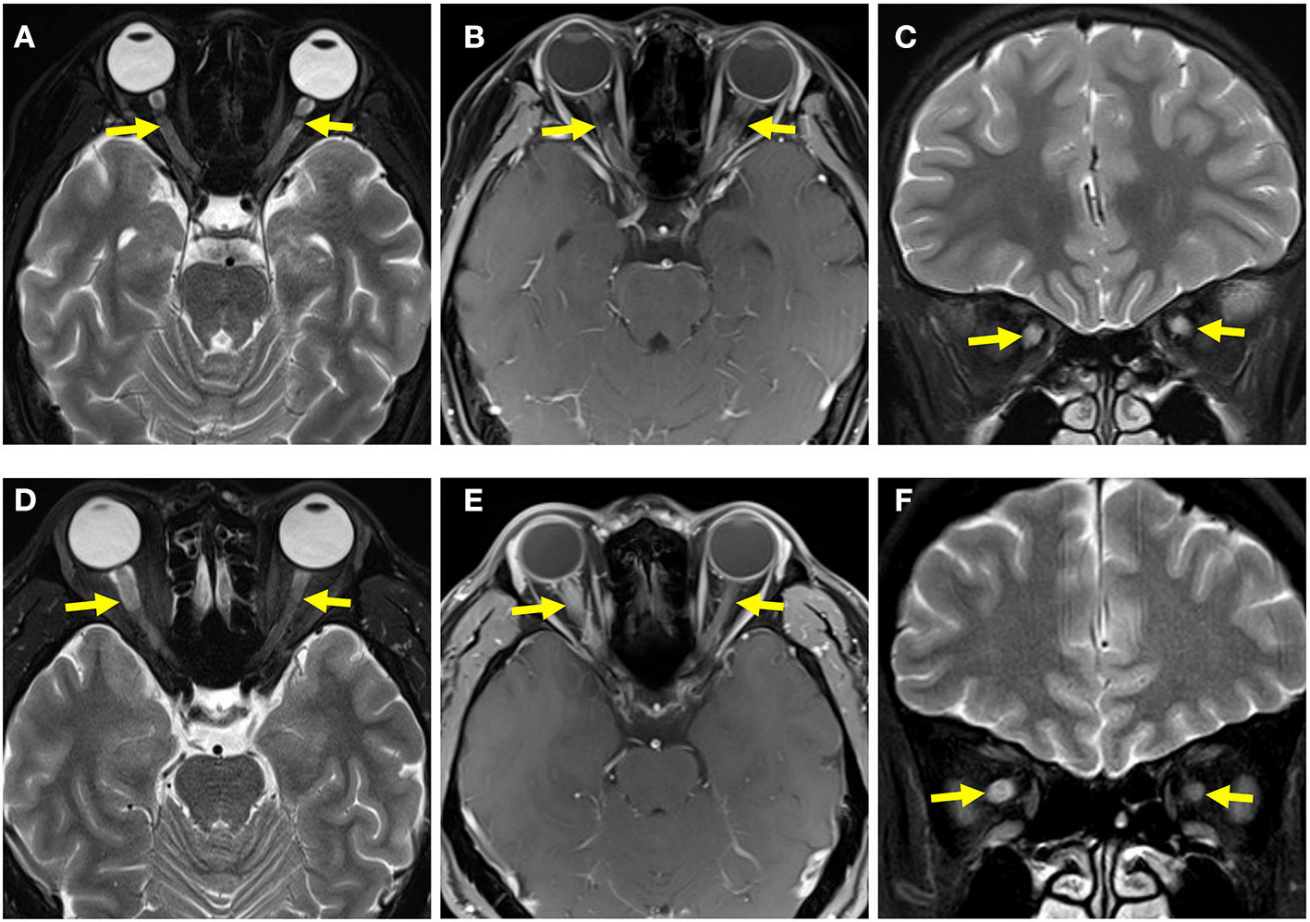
Optic nerve MRI of pediatric and adult patients. (A) T2-weighted MRI of the optic nerve of a pediatric patient showed bilateral optic-nerve thickening. (B) T1-weighted gadolinium MRI of the optic nerve in a pediatric patient showed bilateral optic-nerve enhancement. (C) Coronal image showed bilateral optic-nerve thickening. (D) T2-weighted MRI of the optic nerve of an adult patient showed that the right optic nerve was thicker than the left optic nerve. (E) T1-weighted gadolinium MRI of the optic nerve of an adult patient showed right optic-nerve enhancement. (F) A coronal image showed that the right optic nerve was thicker than the left optic nerve.
